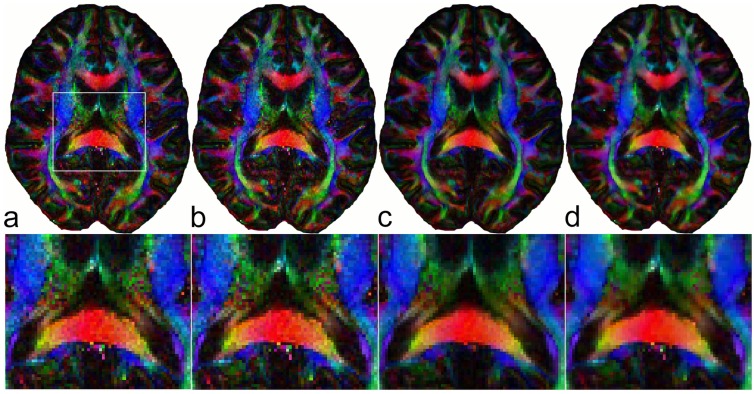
FA map from data obtained using one measurement (first out of three averages) (a), FA map from the denoised set of DWI data (
